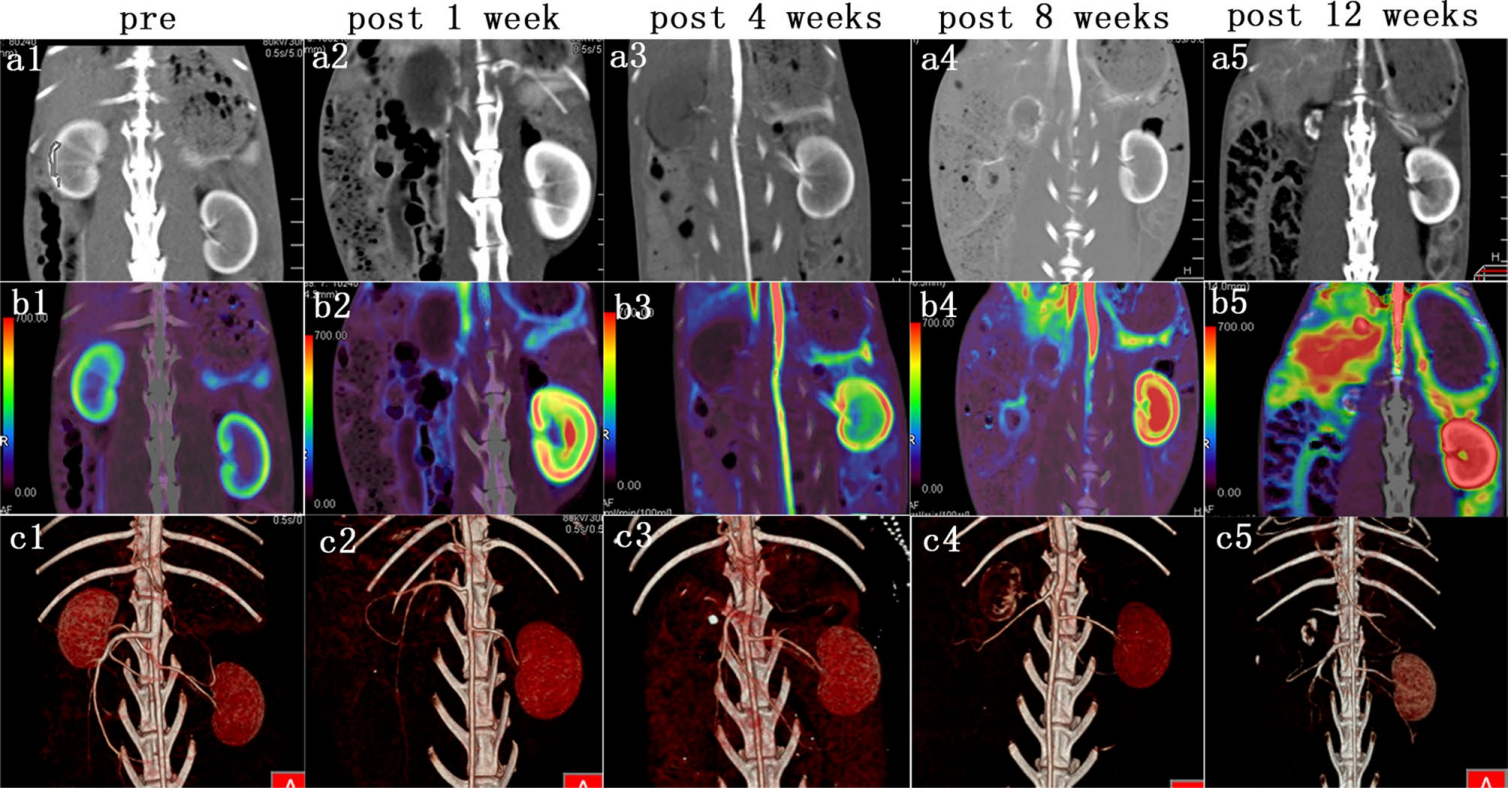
Images of CT examinations before and after embolization at various times. (a1-5) Enhanced images show significant shrinkage of the right kidney, with large calcifications. (b1-5) The perfusion maps show different colors of BF in the right kidney. (c1-5) The VRT images show significant calcification of the right kidney, but the left kidney and large blood vessels are clearly displayed.
